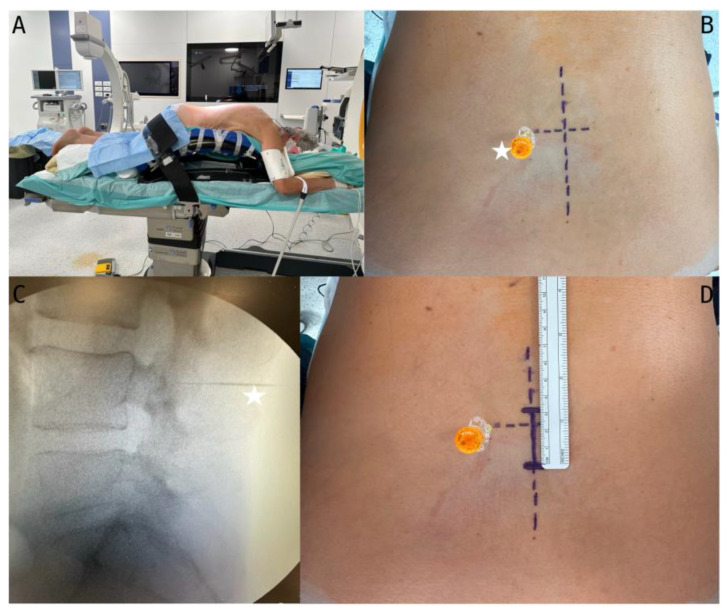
(A) Positioning on a Wilson frame and surgical room setting; (B) spinal needle (white star) positioned laterally on the contralateral articular process of the intended surgical site; the long line corresponds to the midline, and the short line corresponds to the level for L4L5; (C) X-ray control of the correct L4 level; (D) drawn incision extending one-third superior and two-thirds inferiorly to the point level of about 3 cm.
